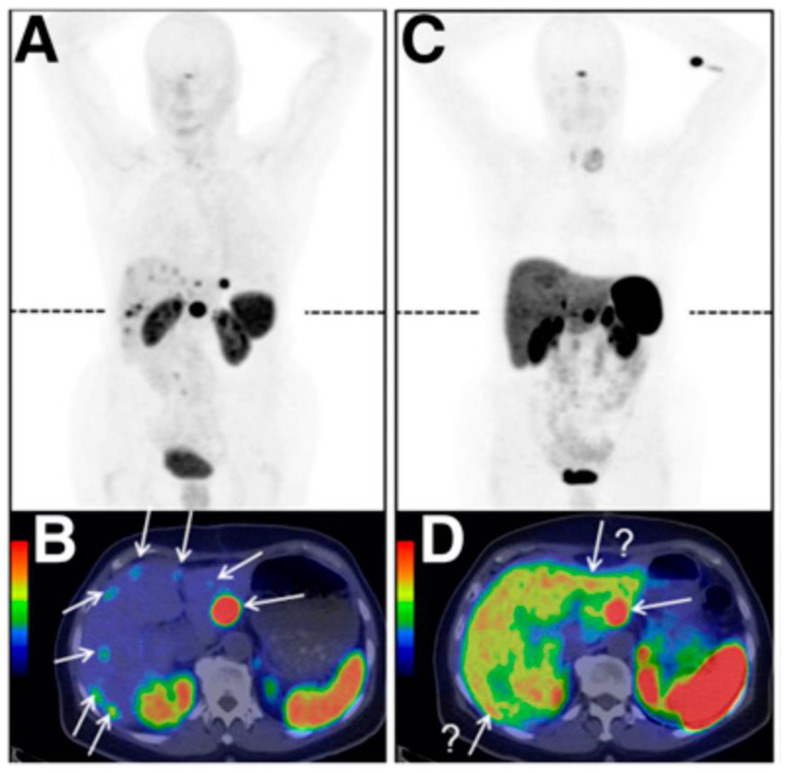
Comparison between [^68^Ga]-OPS202 (A,B) and [^68^Ga]-DOTATOC (C,D) PET/CT images of the same patient with ileal neuroendocrine tumours, showing bilobar liver metastases (from Rangger et al. [233]).
